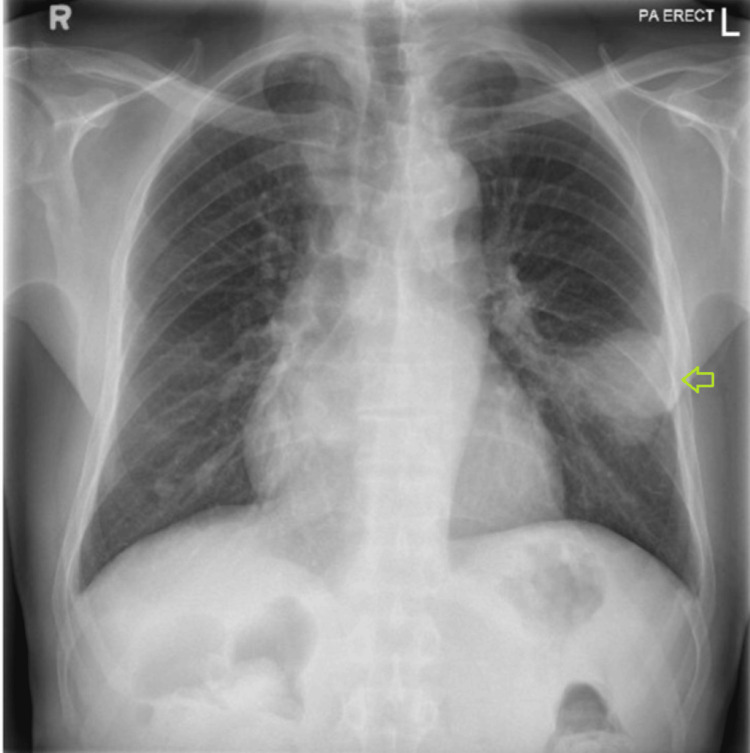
Posterior-anterior chest X-ray showed non-homogeneous opacity in the mid-lower zone of left lung (green arrow).
